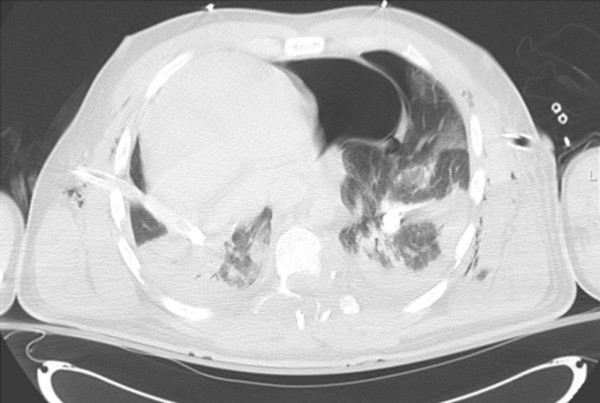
Axial chest CT demonstrating multiple parenchymal lung contusions; collapsed bilateral haemopneumothoraces; tube thoracostomies; surgical emphysema; large left-sided pneumopericardium; and displacement of the heart into the right hemithorax.
